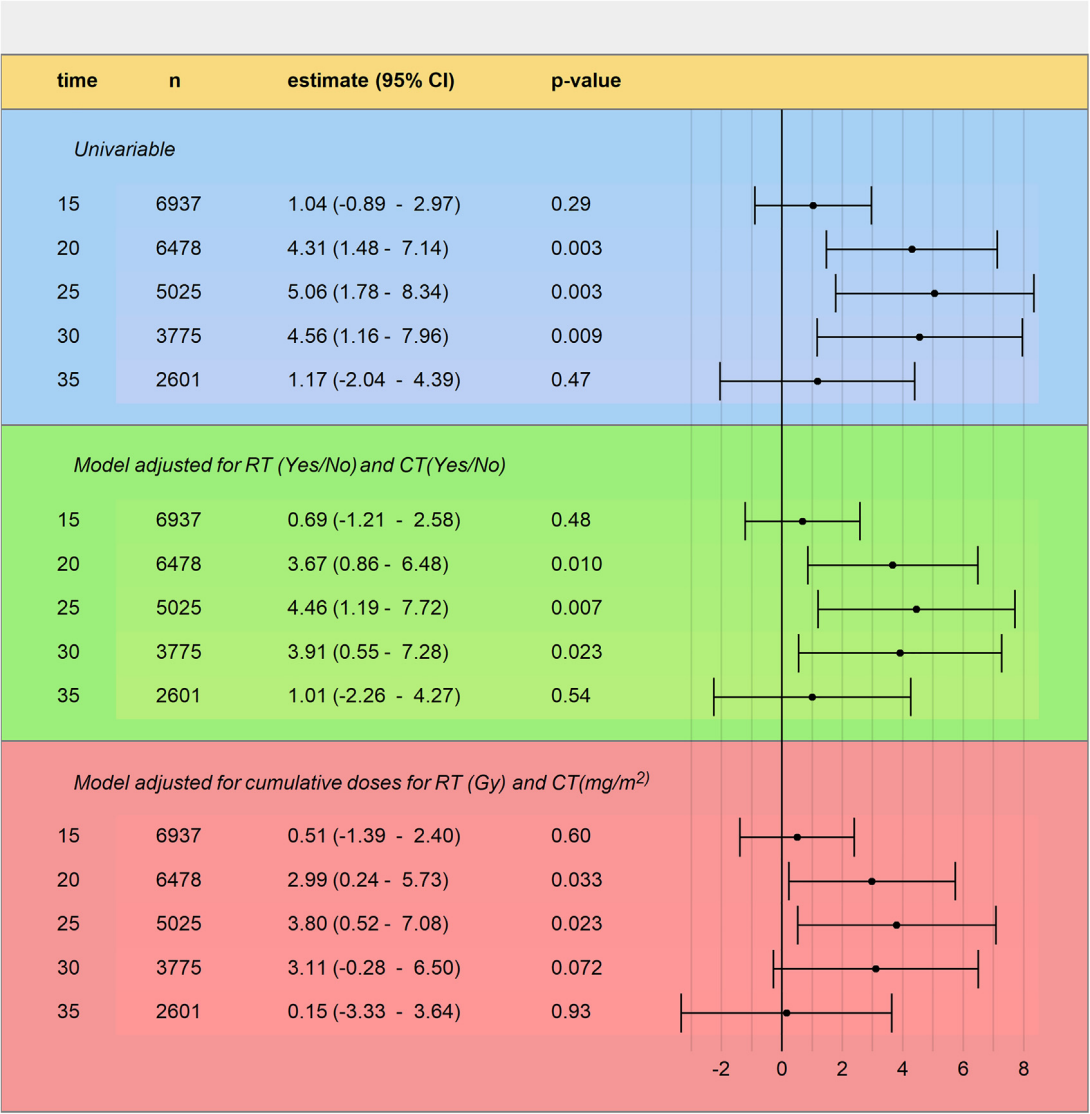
Additive Effect of SMN on Cumulative Incidence of Cardiac Disease This plot summarizes the effect of a second malignant neoplasm (SMN) on the cumulative incidence of cardiac disease. Using an additive model on the cumulative incidence, combined with a landmark strategy, we determined that among patients surviving 25 years after diagnosis, experiencing an SMN was associated with an increased cumulative incidence of cardiac disease of 3.8%. We used time since childhood cancer diagnosis and included death as a competing event. Multivariable models were also adjusted for sex, age, and year of childhood cancer diagnosis. CT = chemotherapy; RT = radiotherapy.
